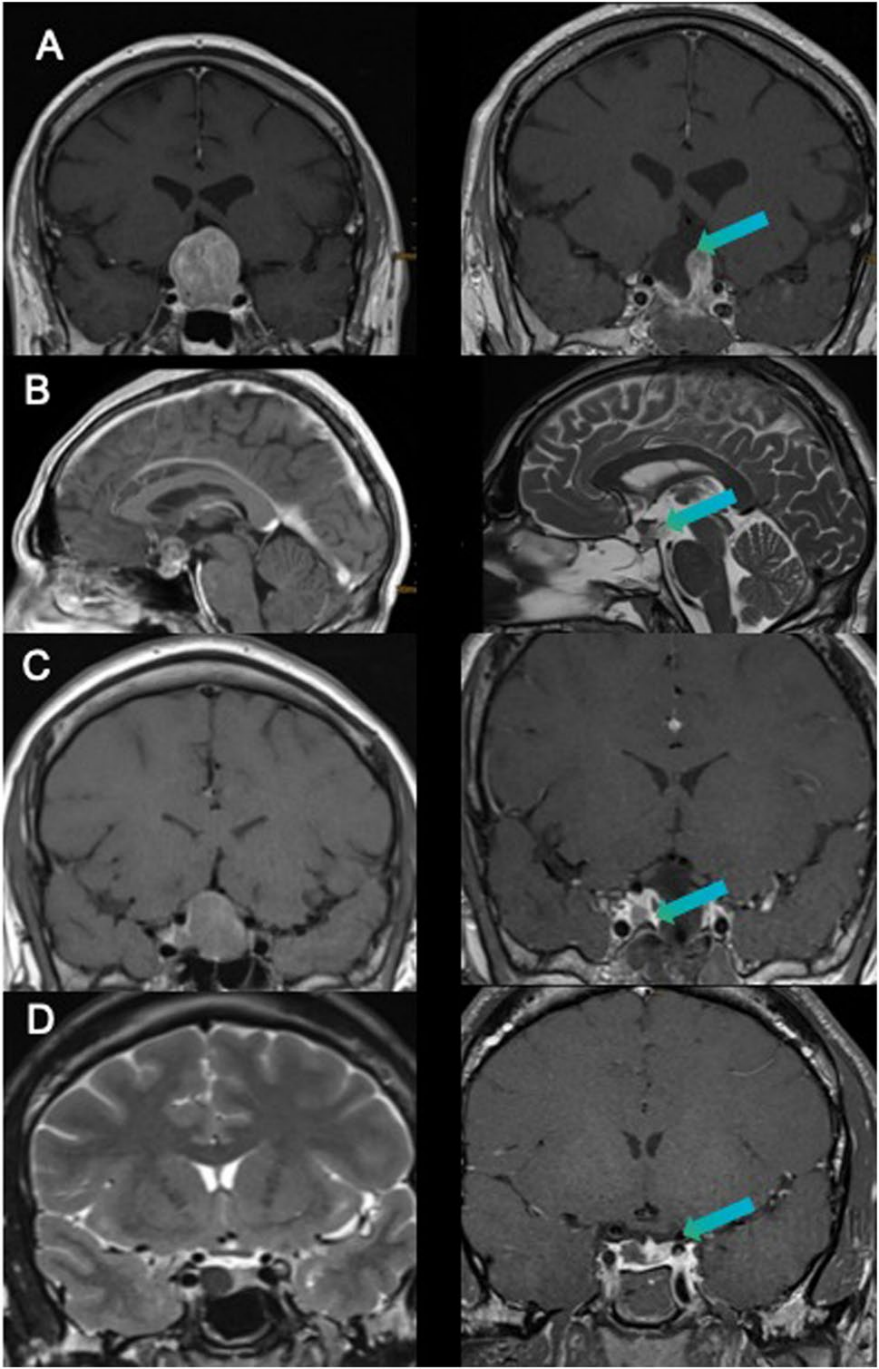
Different location of residual adenomas visible in intraoperative magnetic resonance imaging (A adenoma remnant in diaphragm fold, B intrathecal invasion through the diaphragm, C invasion in the cavernous sinus, D dumbbell-shaped adenoma not visible in preoperative images
